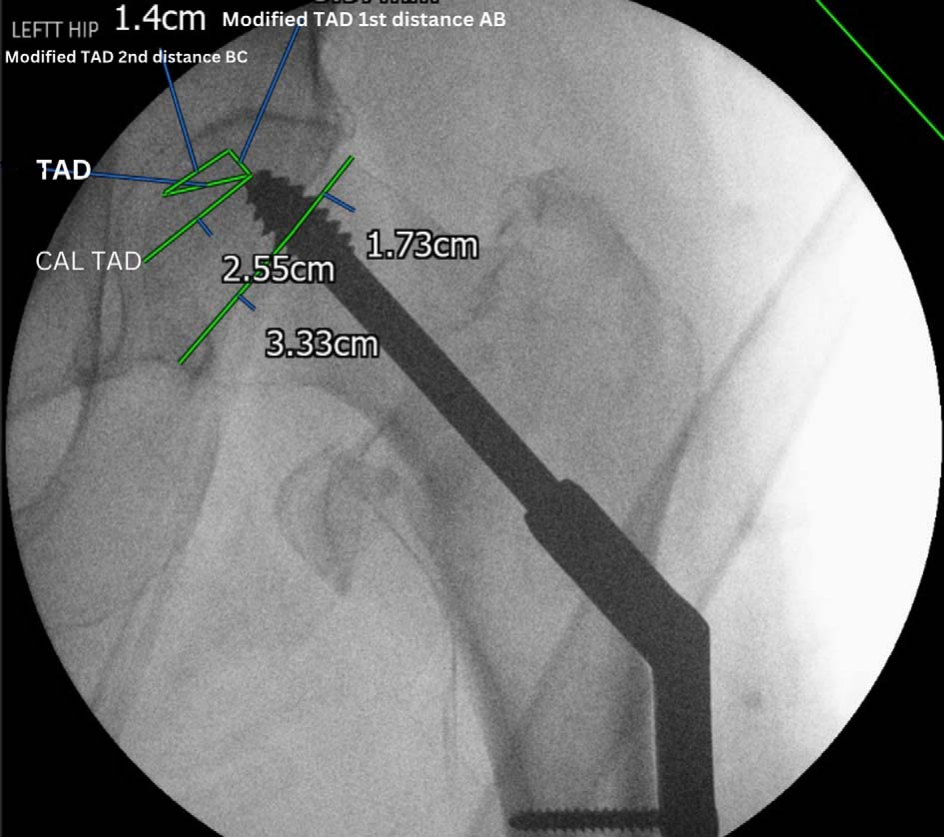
Intraoperative X-ray showing the technique used to measure TAD, Cal TAD, and modified TAD.
